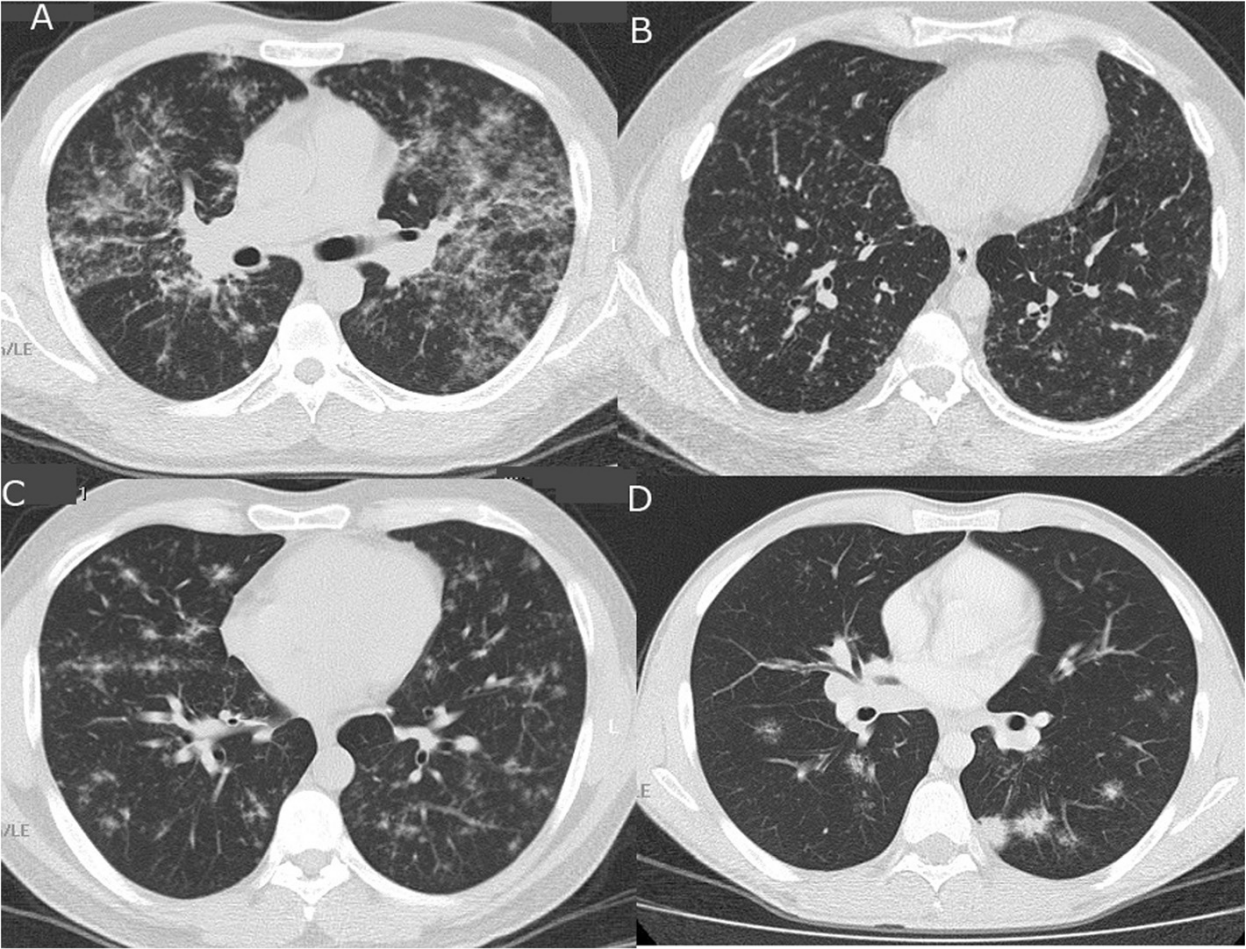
Some HRCT patterns (A: Ground glass opacity, B: Micronodule, C: Nodule, D: Consolidation).
